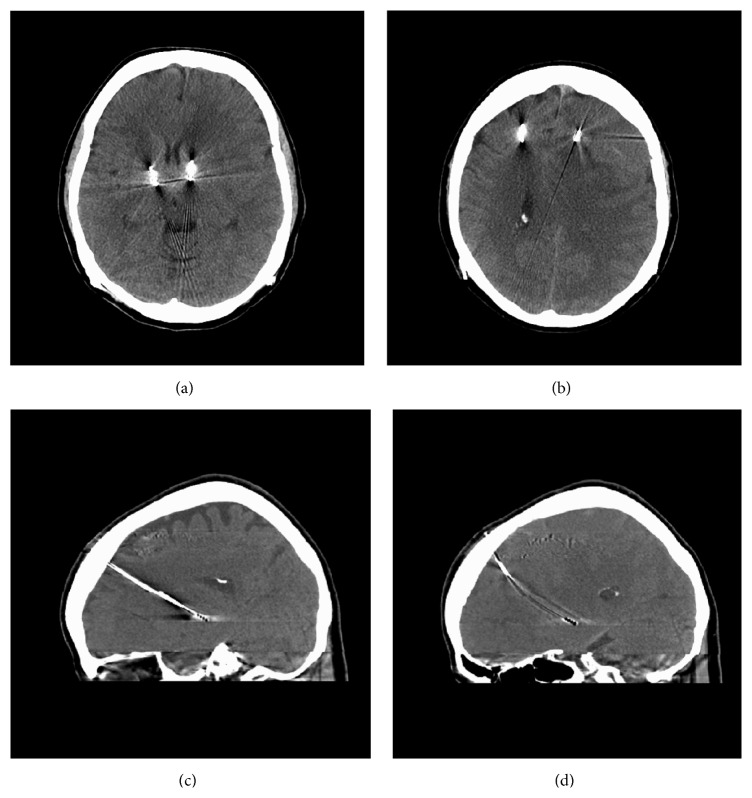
(a) CT head demonstrates left to right brain shift. (b) CT head demonstrates isodense pathology along left convexity. (c) CT head sagittal view demonstrates R DBS lead with relative normal position. (d) CT head sagittal view demonstrates L DBS lead that has bowed compared to the R DBS lead.
